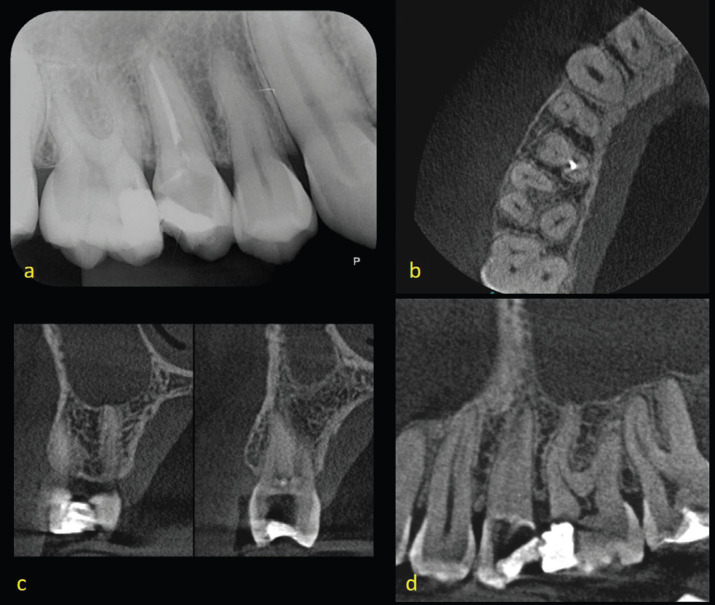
Analized images: radiograph and slices of CT scans of the same region. (a) radiograph, (b) axial, (c) parasagittal, (d) sagital.
